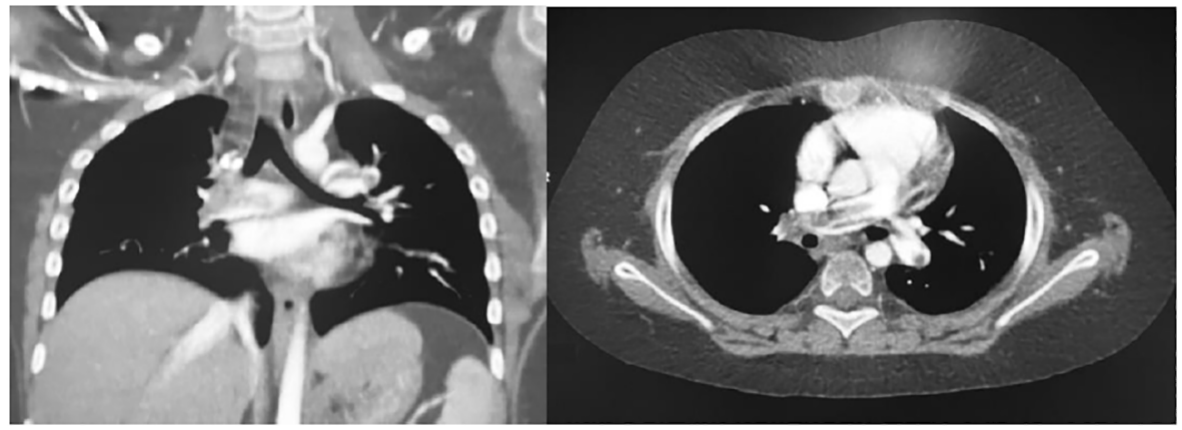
Coronal and axial chest CT images of Pulmonary embolism in patient 12.
